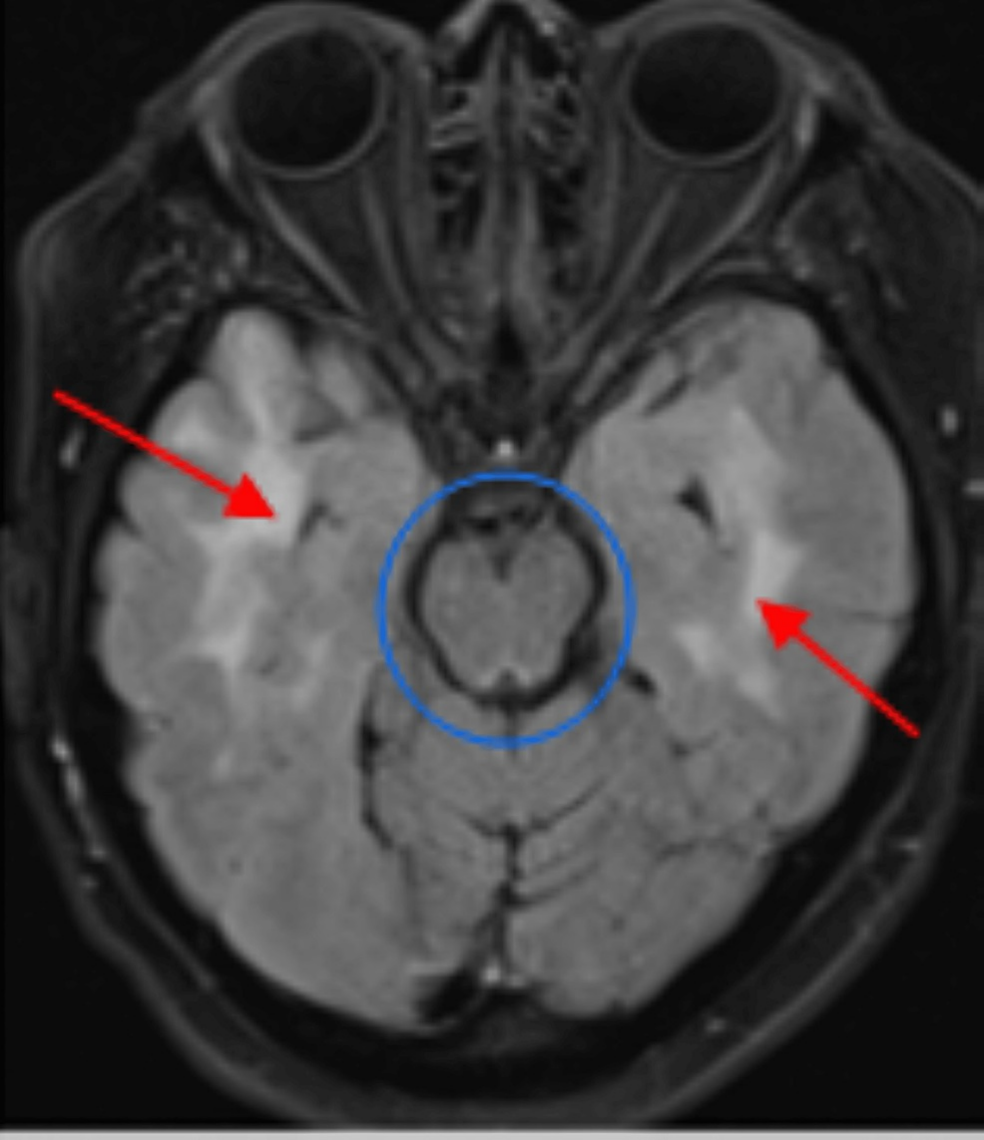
MRI FLAIR sequence showing white matter signal abnormality (red arrows) sparing midbrain (blue circle). FLAIR: fluid-attenuated inversion recovery.
